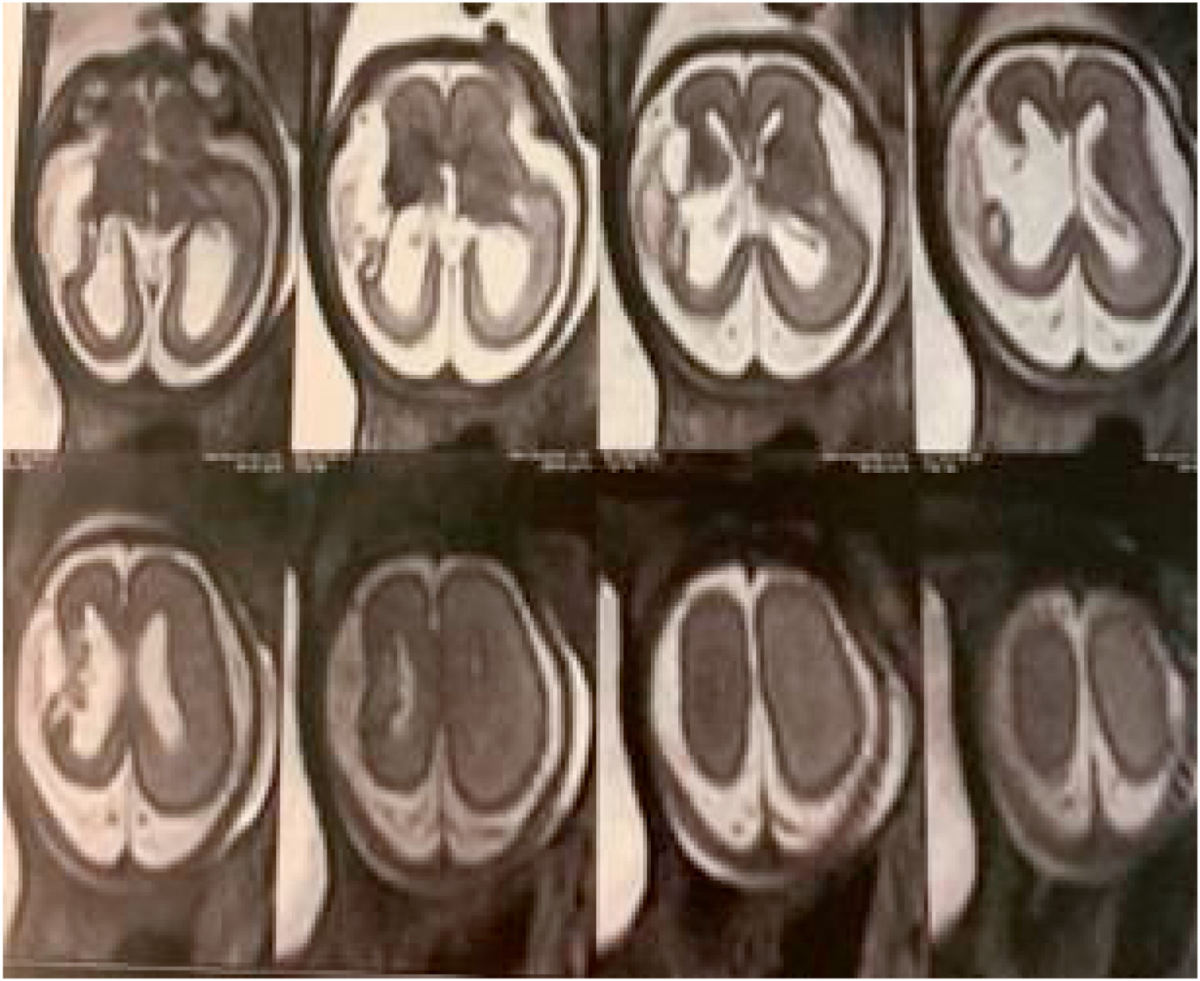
Fetal cerebral MRI. Fetal MRI scan (25 weeks of gestation) showing extensive poroencephaly (2.3 cm of diameter) in the fronto- temporal and insular zone with “ex vacuo” enlargement of the right lateral ventricle, reduced volume of the right cerebral hemisphere. An anomalous development of the middle-lower right frontal cortex is highlighted, which shows a polylobulated aspect due to reduced amplitude of the furrows and increased number of turns (suspect of micropolygyria) extended up to the right parietal lobe.
